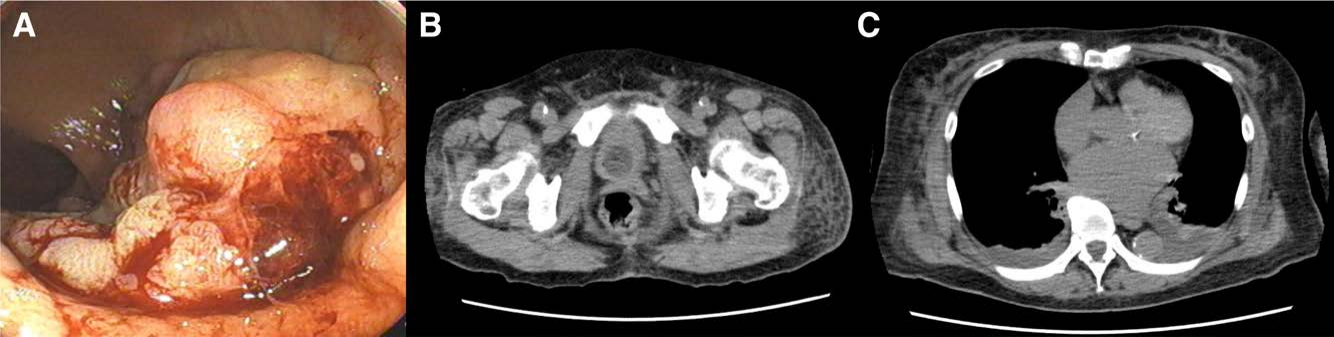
Representative images. (A) Colonoscopy image showing a type 2 tumor in the lower rectum. (B) Computed tomography scan reveals rectal wall thickening with contrast enhancement. (C) Bilateral pleural effusion is observed.
